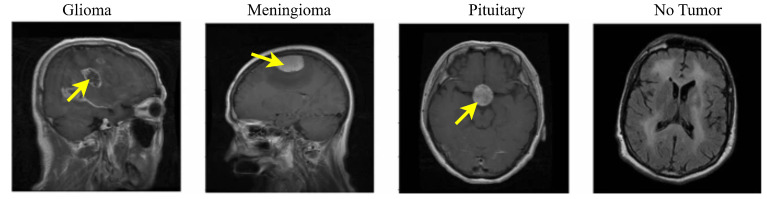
Class-wise representative MRI samples from the dataset showing Glioma, Meningioma, Pituitary, and No Tumor cases across different anatomical planes. Yellow arrows indicate the presence and location of tumor regions within each MRI class, highlighting the affected areas for improved visual interpretation and class-wise distinction.
